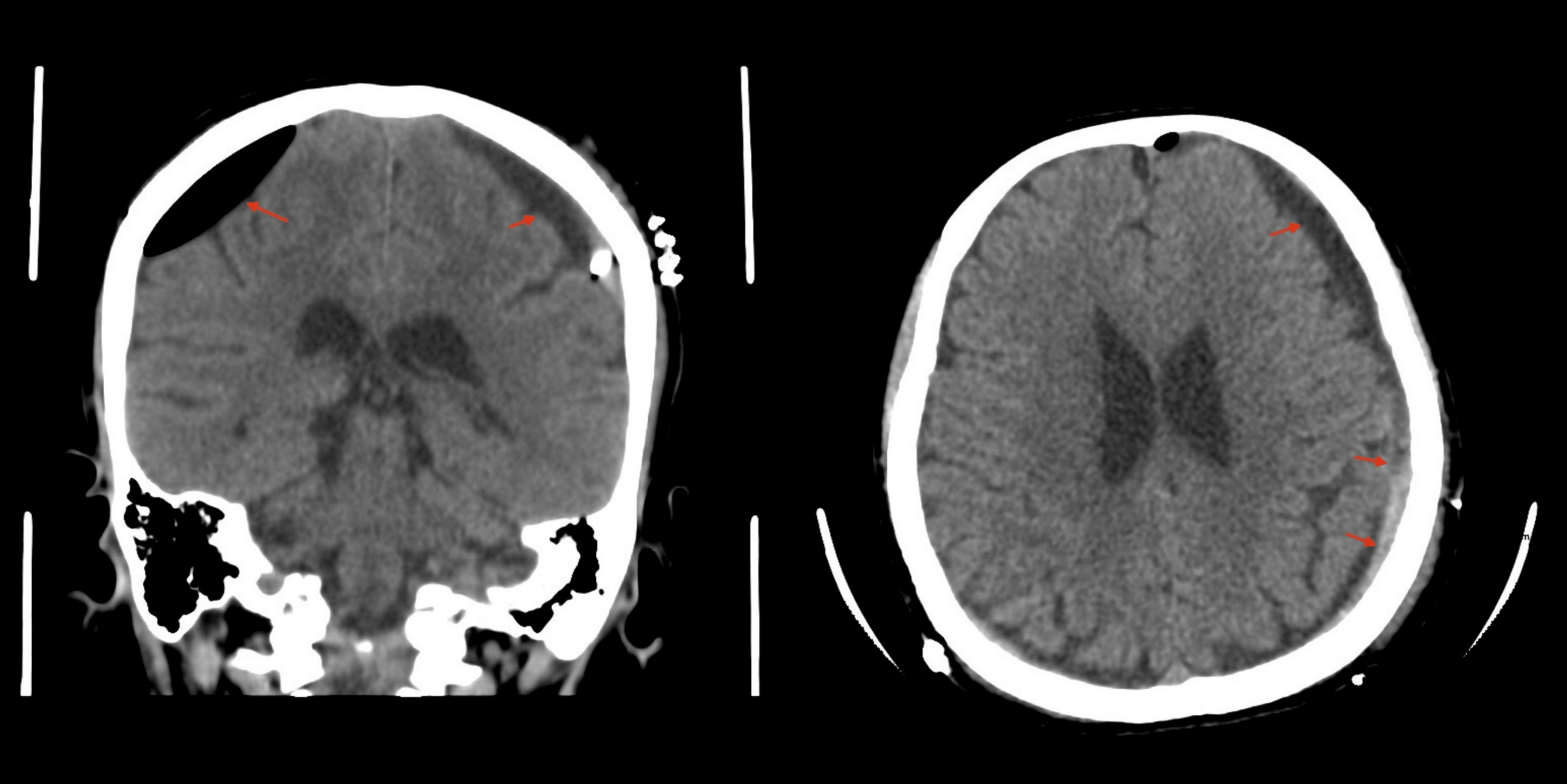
Brain CT post-burr hole evacuation. Postoperative axial and coronal brain CT scans showing subdural hemorrhage with a maximum thickness of 0.4 cm on the left side and 0.7 cm on the right side.
